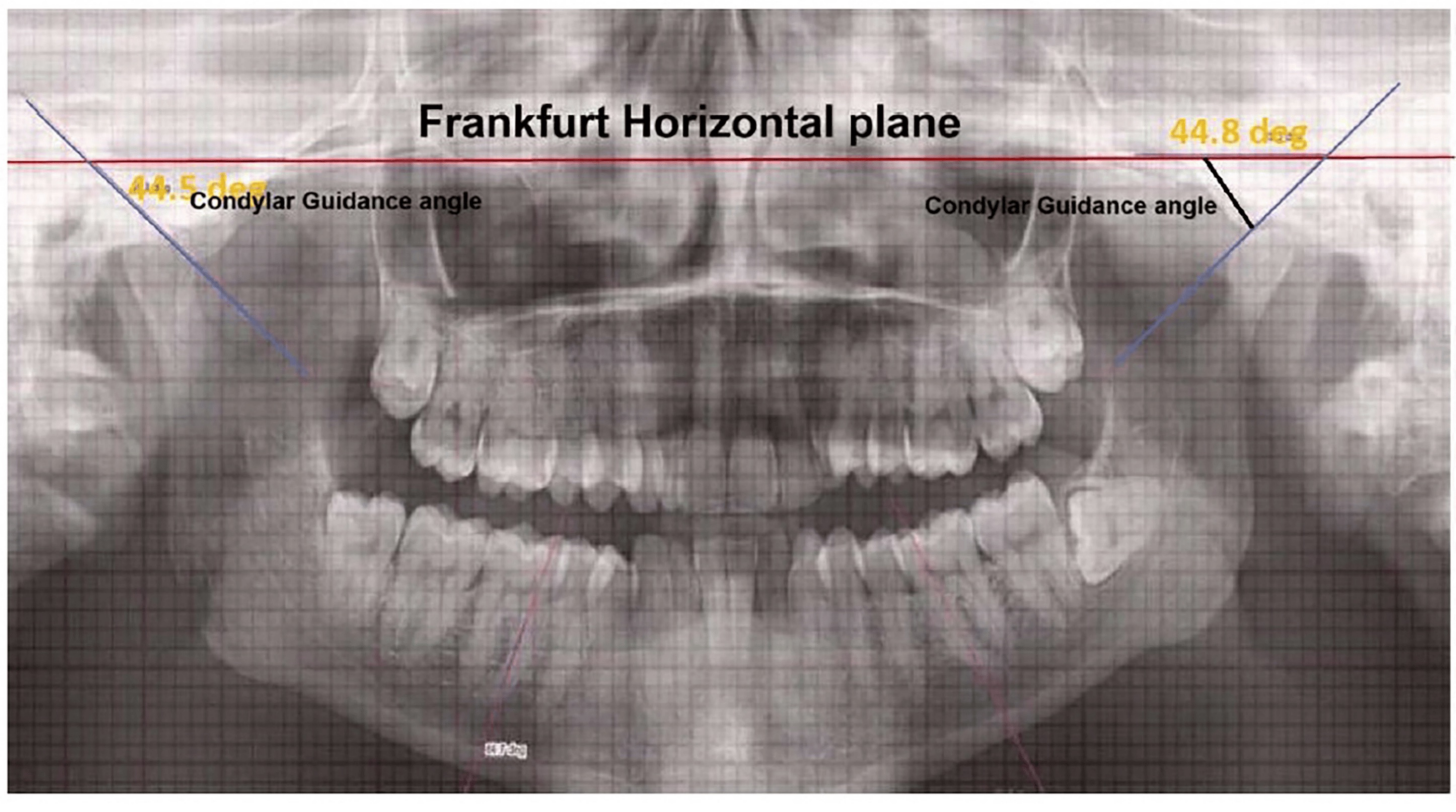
Condylar guidance (CG) – the angle between the Frankfurt Horizontal (FH) plane and constructed condylar path on orthopantomogram (OPG)
